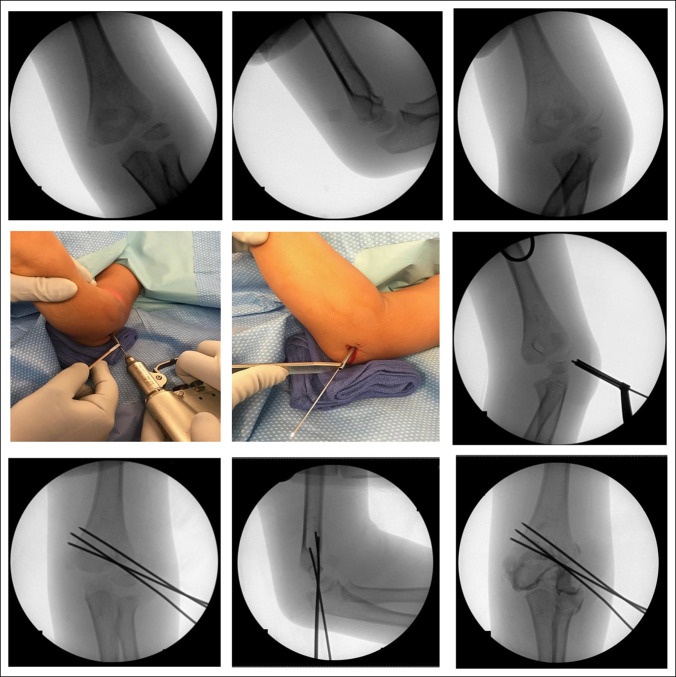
Fluoroscopy showing martini modification: A–C, AP, lateral, and oblique radiographs showing displaced and rotated lateral condyle fractures. D, 0.062-inch Kirschner wire placed into the lateral condyle fragment with a toothed drill over the wire for manipulation and stabilization. E and F, AP and lateral radiographs showing bicortical fixation with three Kirschner wires. G, AP arthrogram confirming acceptable reduction of the lateral condyle.
